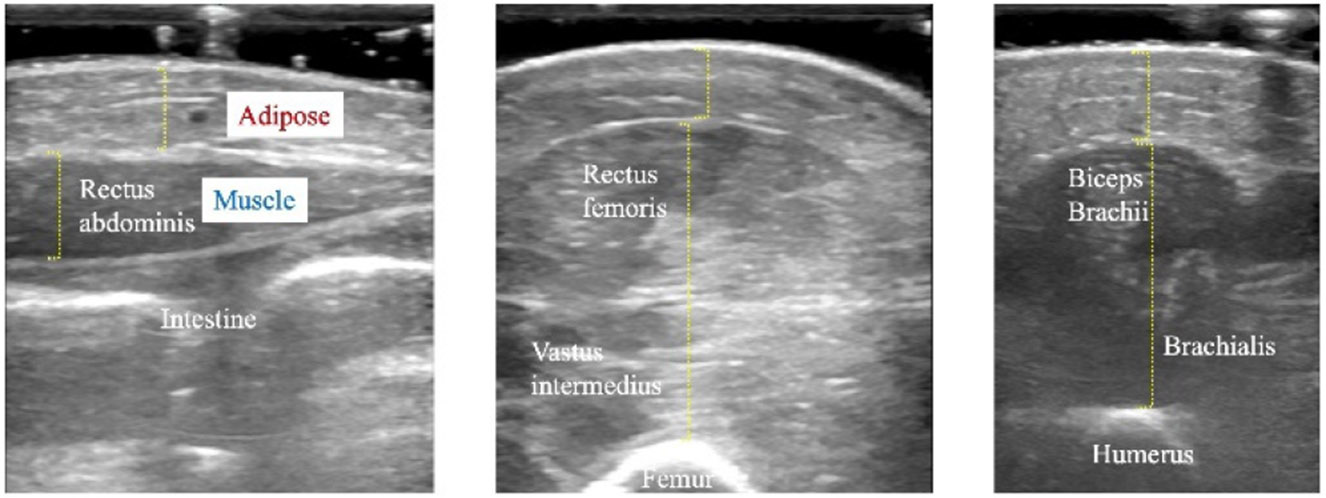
Clinical ultrasound image measurement of abdomen, biceps, and quadriceps of the premature infant. *Left*: abdomen, *Middle*: biceps, and *Right*: quadriceps.
